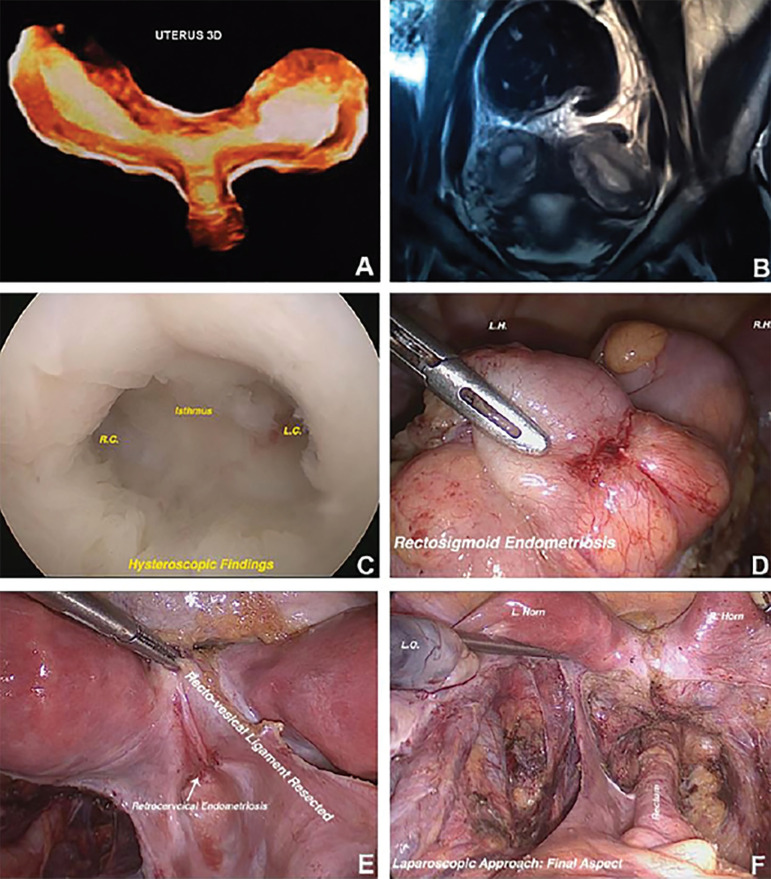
A) View of uterus in 3D ultrasound. B) View of uterus in magnetic resonance imaging (MRI). C) Hysteroscopic view of uterus. D) Rectosigmoid endometriosis. E) Recto-vesical ligament resected. F) Final aspect after laparoscopic approach. RC: Right Horn; LR: Left Horn; LO: Left Ovary
